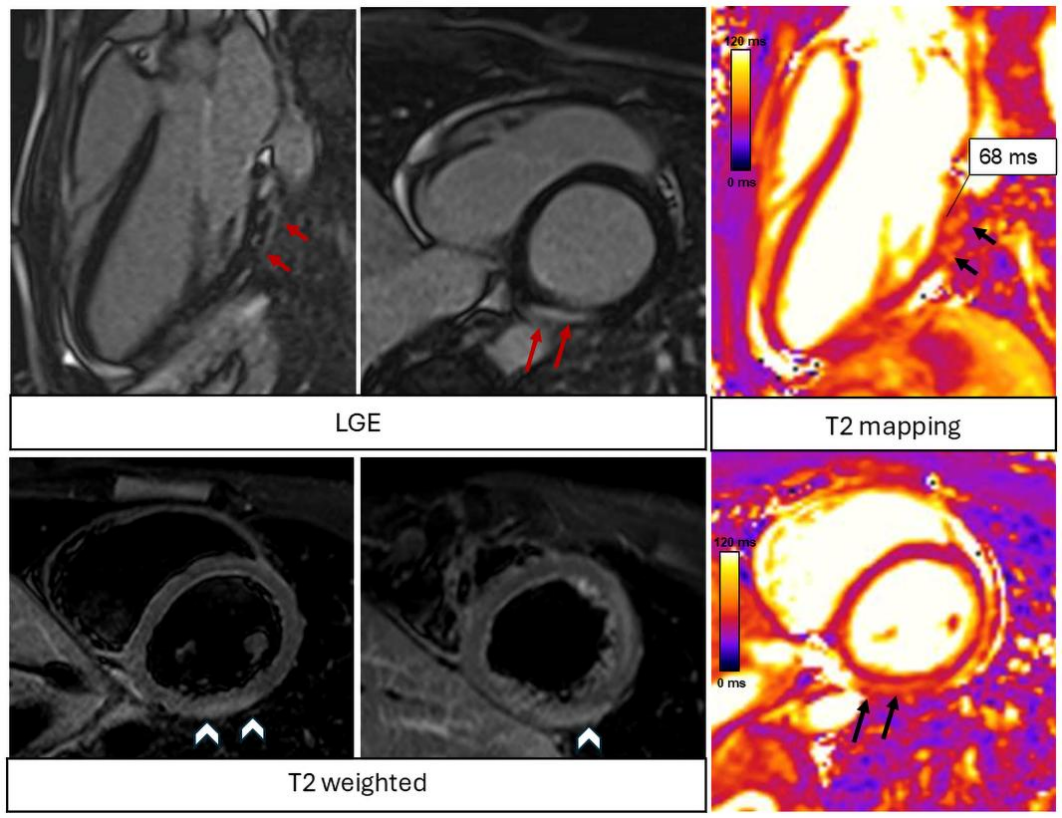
Cardiac magnetic resonance images of a patient with acute myocarditis. At basal infero-lateral wall, there is evidence of increased signal intensity with a subepicardial pattern in both T2-weighted and LGE images in keeping with the diagnosis of acute myocarditis. Increased T2 mapping values in the same areas confirm the presence of myocardial oedema. LGE, late gadolinium enhancement.
